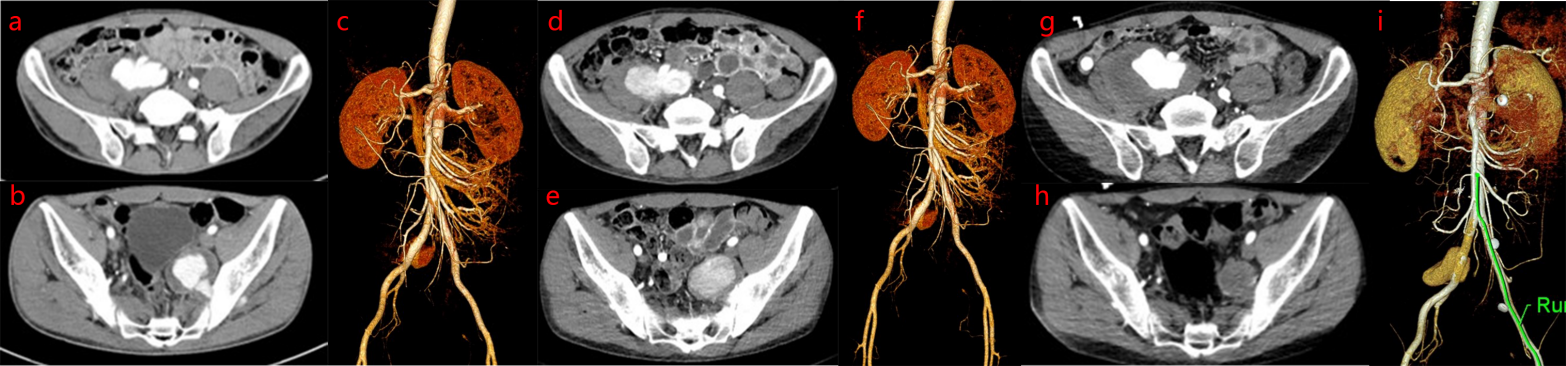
Abdominal computed tomography angiography (CTA) examination. Abdominal CTA images show dynamic progression of pseudoaneurysm based on drug intervention before bare stent implantation: the area of pseudoaneurysm of the right CIA is larger, while the area of the pseudoaneurysm of the left IIA is smaller. (A-C). On admission abdominal CTA revealed rupture of the right common iliac artery (CIA) and left internal iliac artery (IIA), which was complicated by the formation of a pseudoaneurysm. (D–F). After 1 month of drug therapy, abdominal CTA revealed that the area of the pseudoaneurysm of the right CIA was larger than that at the first examination, while the area of the pseudoaneurysm of the left IIA was similar to that at the first examination. (G–I). After 10 months of drug therapy, abdominal CTA revealed that the area of pseudoaneurysm of the right CIA was larger than that at the second examination, while the area of pseudoaneurysm of the left IIA was smaller than that at the second examination.
